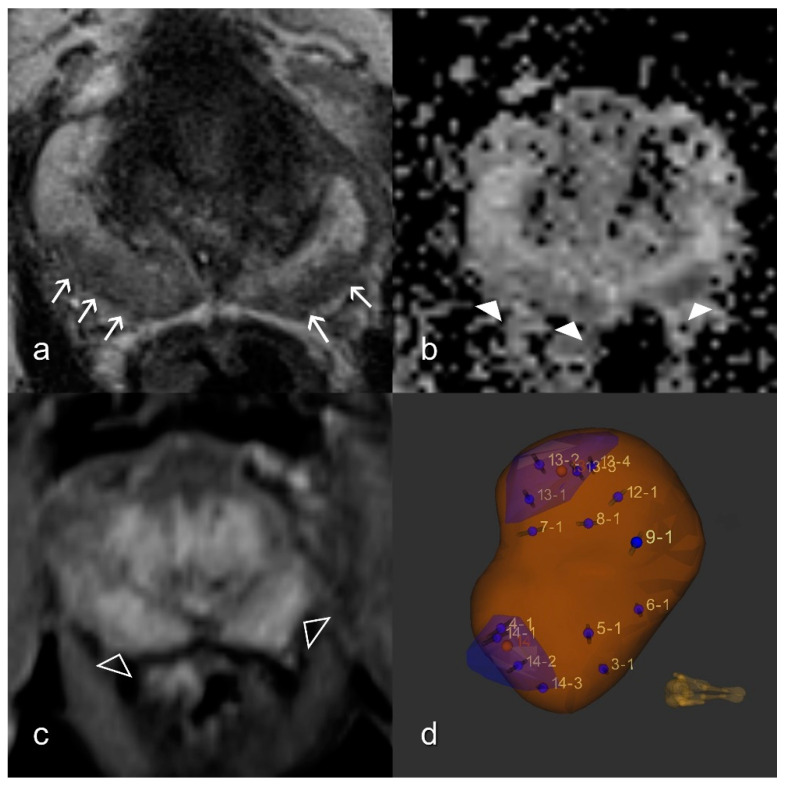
Reference standard. Imaging findings showing patchy T2W signal attenuation (white arrows) in the peripheral zone on both sides (a), moderate reduction (white arrowheads) in the ADC map (b), and diffuse enhancement (empty arrowheads) in the dynamic T1W VIBE (c); Formally, this represents the PI-RADS 3 constellation; (d) The MRI/ultrasound (US)-guided biopsy 3D model depicts the target lesions in the blue zones and the template biopsy cores (numbered). Pathology confirmed no malignancy. ADC, apparent diffusion coefficient; T1W, T1-weighted; T2W, T2-weighted; VIBE, Volumetric interpolated breath-hold examination.
